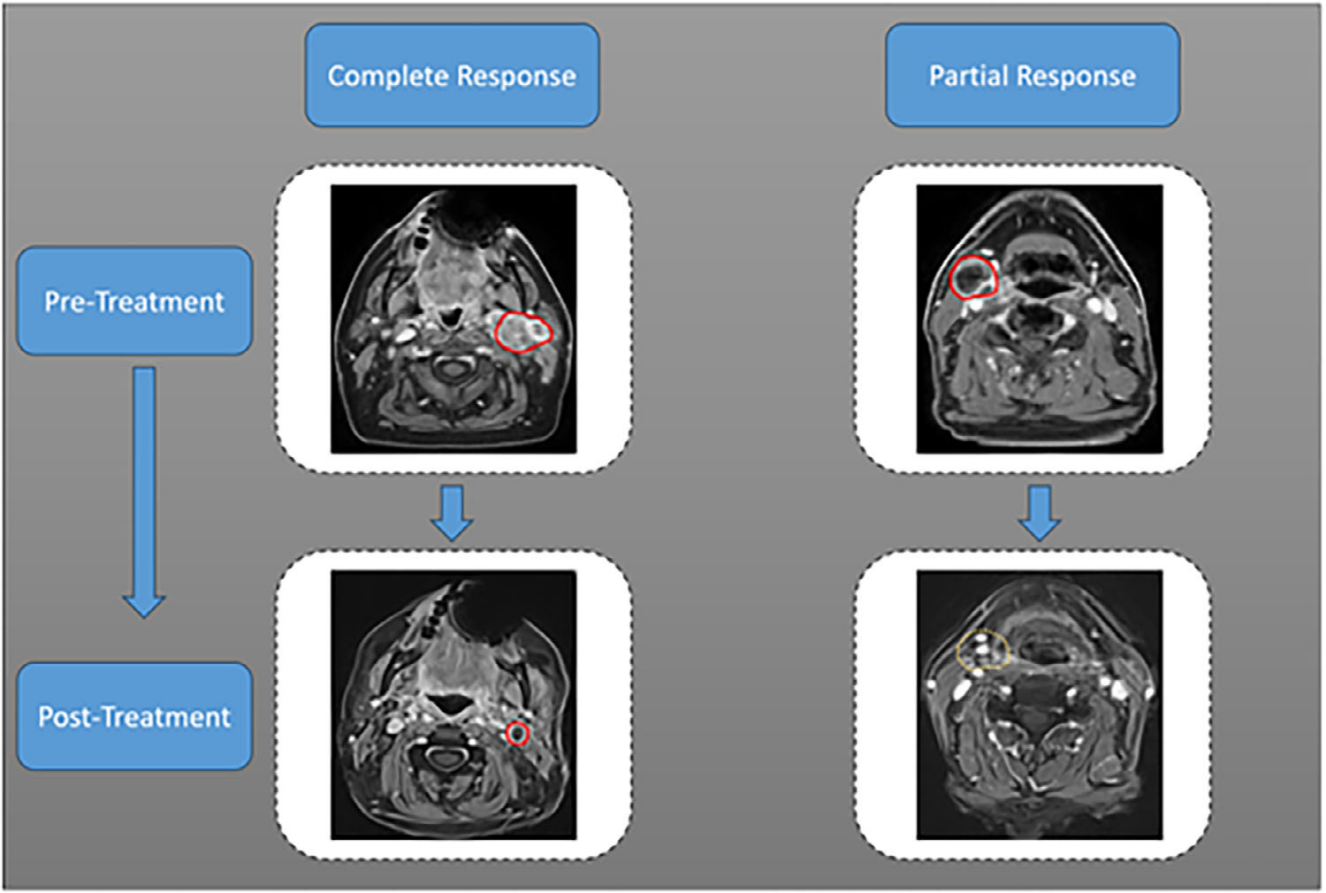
Pretreatment (before radiation therapy) and posttreatment (after radiation therapy) of axial T1‐weighted MRI images for a patient with complete response (CR) and a patient with partial response (PR).
